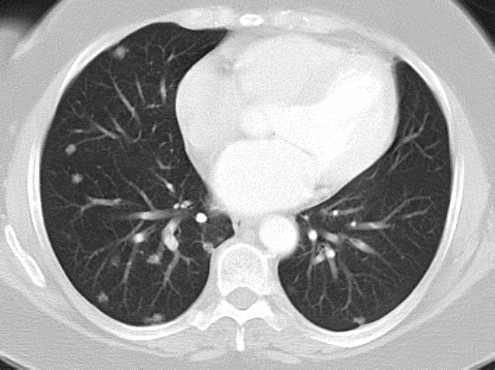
Computed tomography scan of the thorax performed at presentation in 2002 demonstrating multiple pulmonary metastases.
